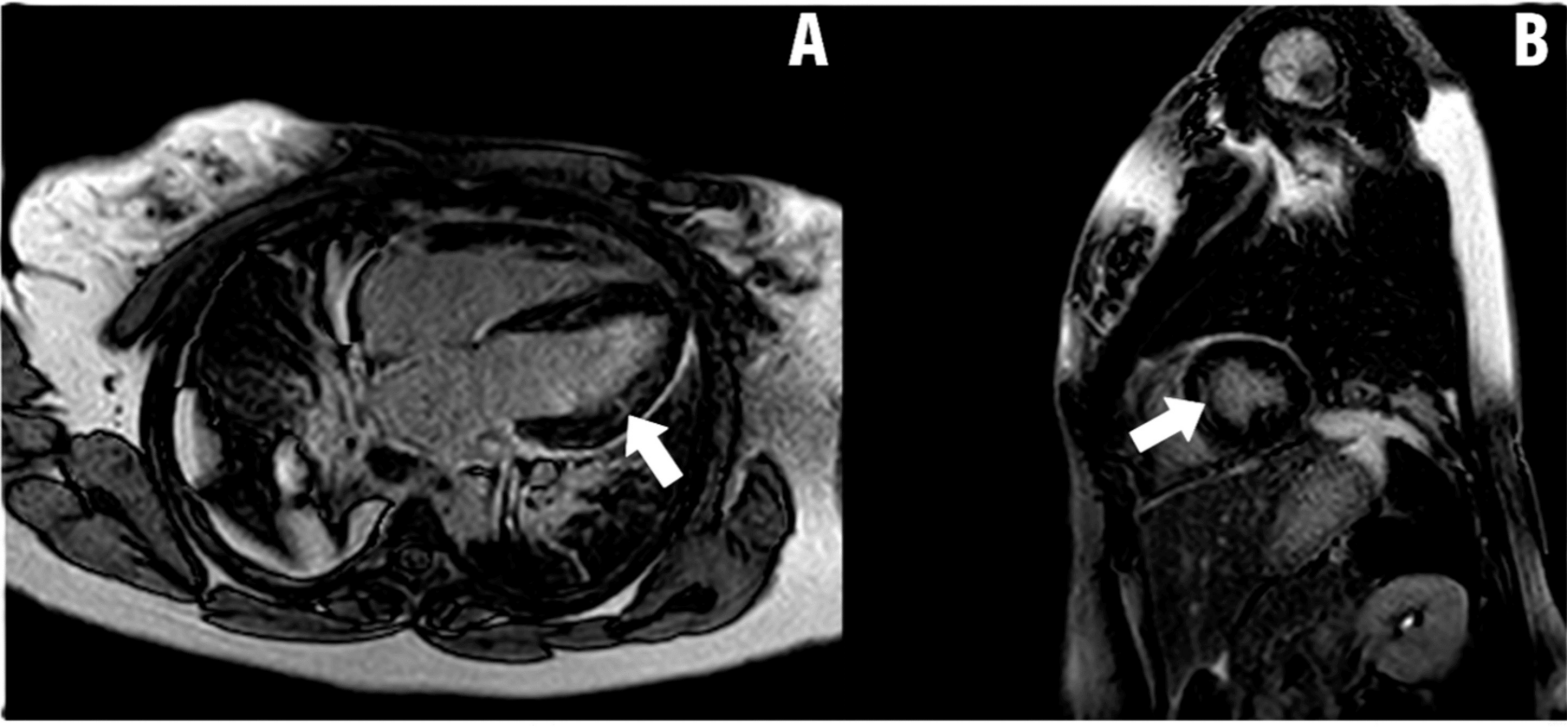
Cardiac MRI reveals both pericarditis and myocarditis. Both arrows point to heterogeneous mild myocardial delayed enhancement along with circumferential pericardial enhancement. Parametric mapping showed elevated myocardial native T1 time of 1154 ms and myocardial T2 time of 66 ms, consistent with acute perimyocarditis. (A) Horizontal long axis four-chamber anterolateral wall view; (B) Short axis view Image credit: Mark Zenker
